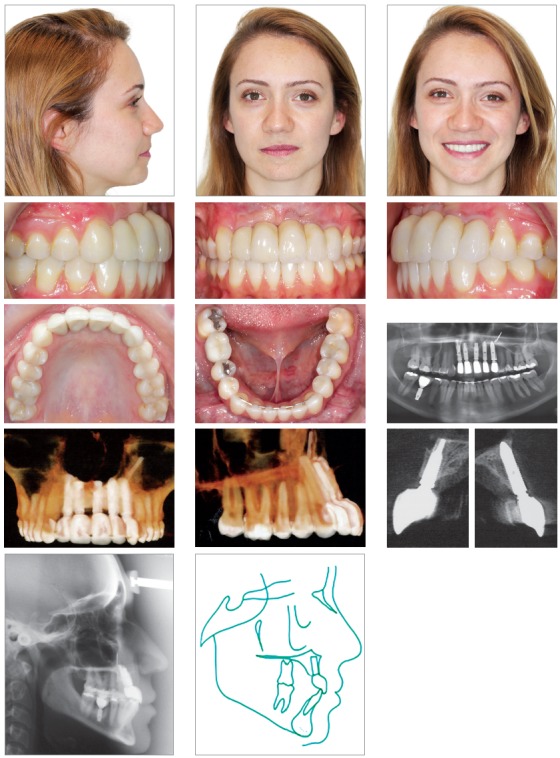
Two-year follow-up photographs demonstrating the maintenance of occlusal stability. CBCT with the implant position.
